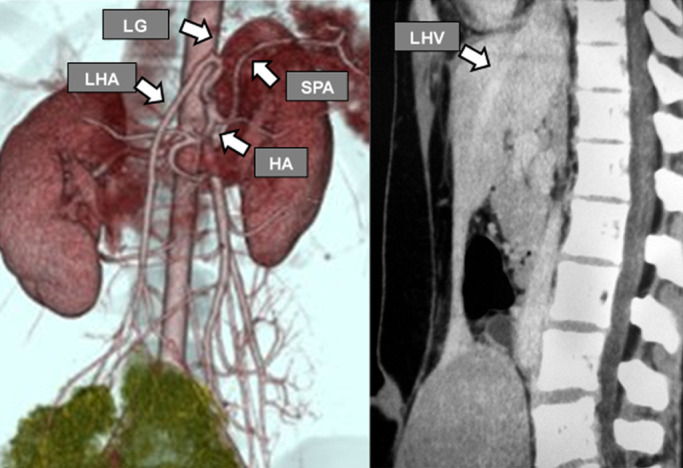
Dynamic CT angiogram The tumor was infused with continuous arteries from the left hepatic artery, and the vein
